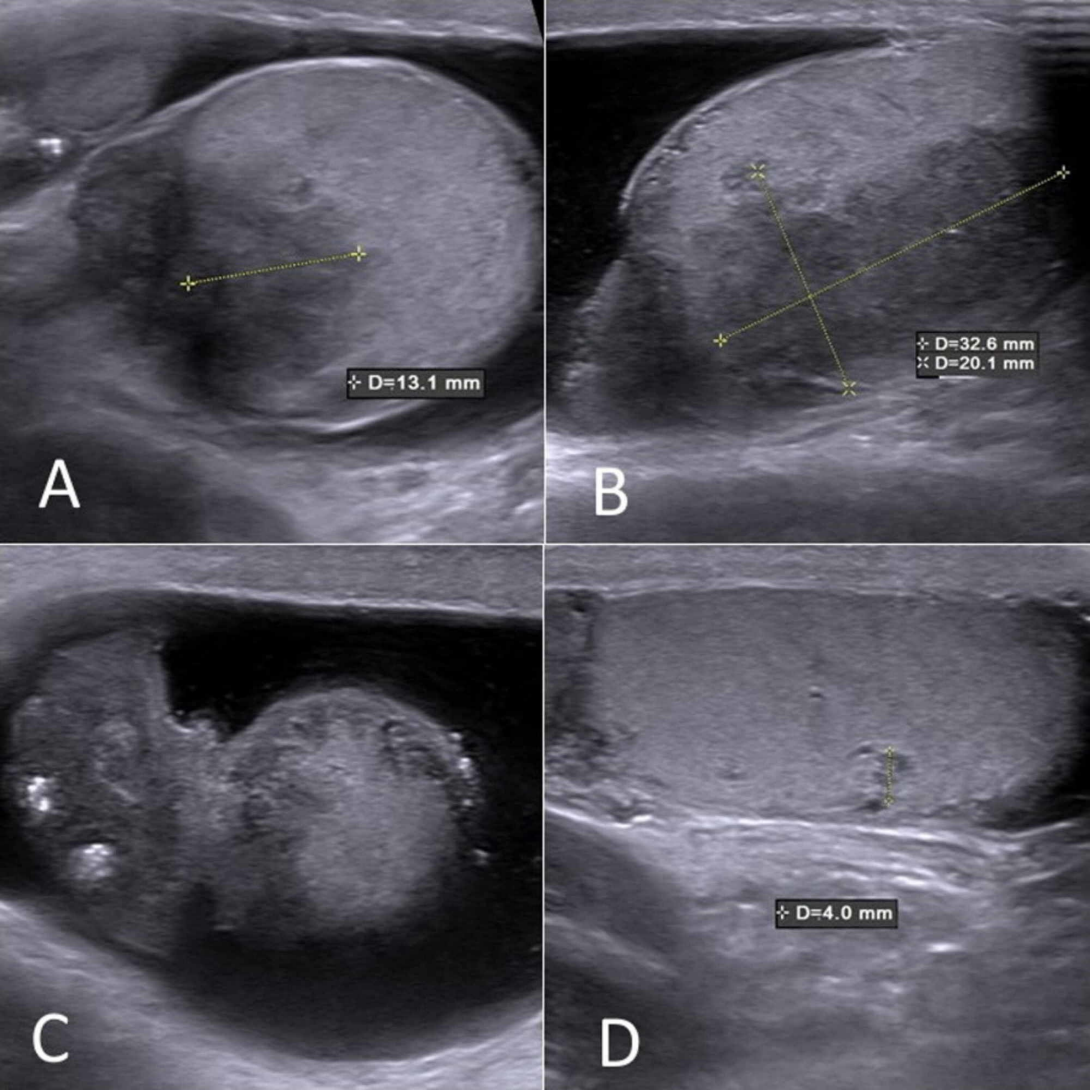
Scrotal ultrasound Bilateral hypoechogenic nodules in both epididymal heads (A, B), and a right-sided testicular infiltration along the rete testis (C). A small parenchymal lesion was noted in the right testis (D).
